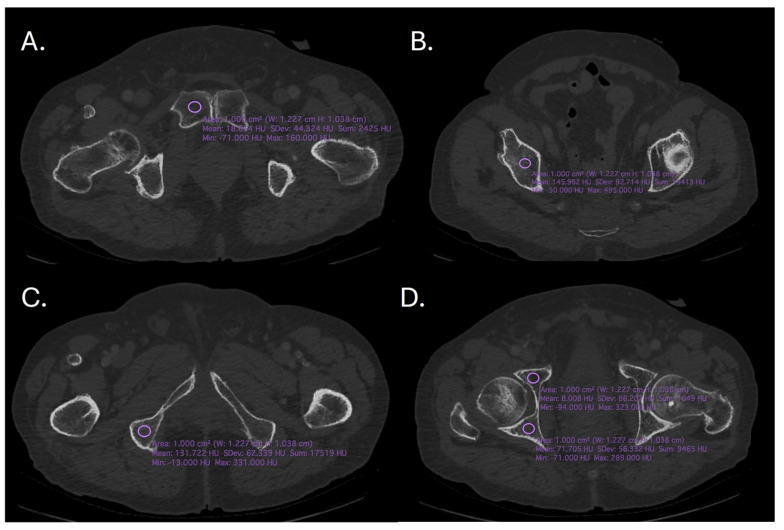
The CT images showing the region of interest (A). Right pubic symphysis, (B). Supracetabular (C). Ischial tuberosity, (D). Anterior and posterior acetabulum.
